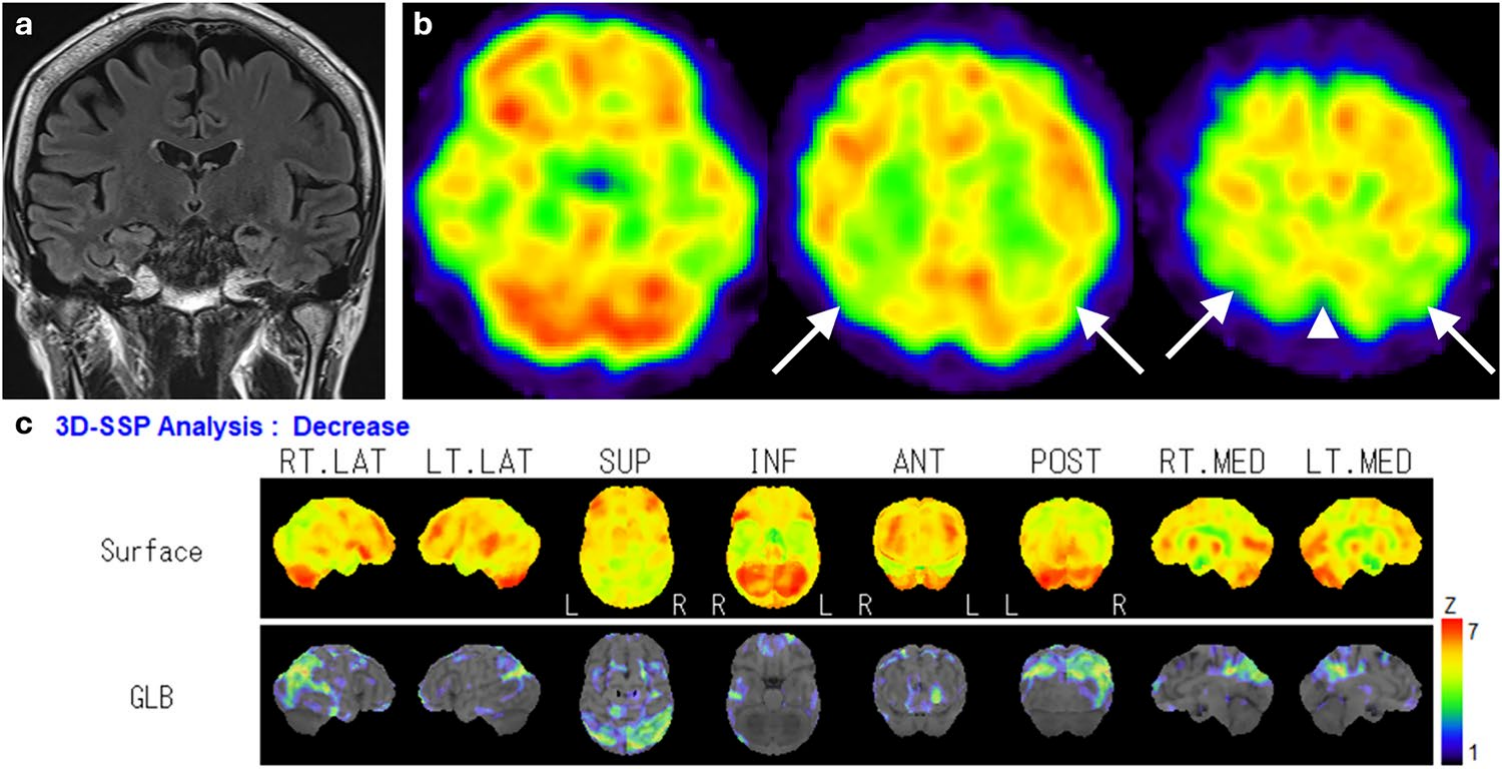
A representative case of AD, a 62-year-old female. a Coronal fluid-attenuated inversion recovery (FLAIR) images showed only mild medial temporal lobe atrophy. b However, IMP brain perfusion images revealed hypoperfusion in the posterior cingulate gyrus to precuneus (arrowhead) and temporoparietal cortex (arrows) compared with the perirolandic cortex and cerebellum. c 3D-SSP analysis demonstrated a similar pattern of hypoperfusion
